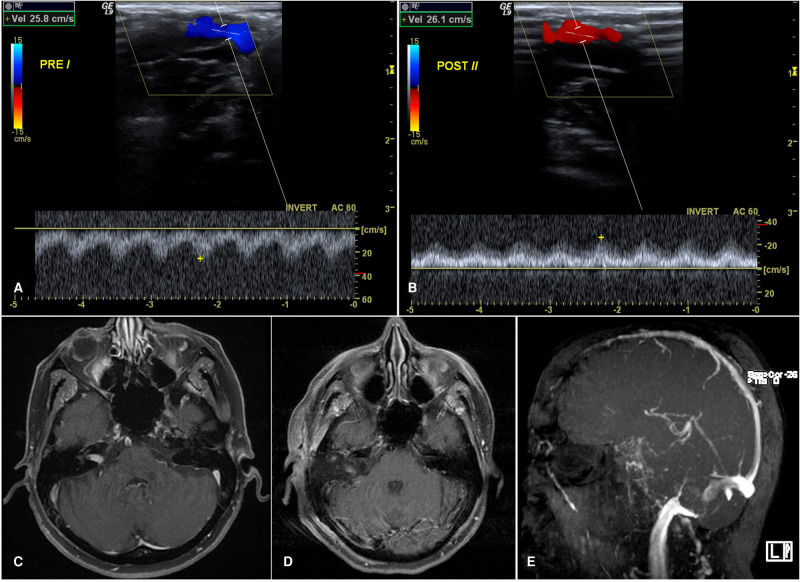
69 year-old female with a right intracanalicular vestibular schwannoma who underwent a translabyrinthine approach for resection due to worsening of clinical symptoms. (A) Doppler ultrasound of the sigmoid sinus prior to dural opening. (B) Doppler ultrasound of the sigmoid sinus prior to closure. (C) Pre-operative MRI brain with contrast demonstrating an intracanalicular vestibular schwannoma. (D) Post-operative MRI with contrast and fat saturation showing a gross total resection of the vestibular schwannoma. (E) Post-operative MRA-TOF of the brain with significant stenosis of the right sigmoid sinus.
